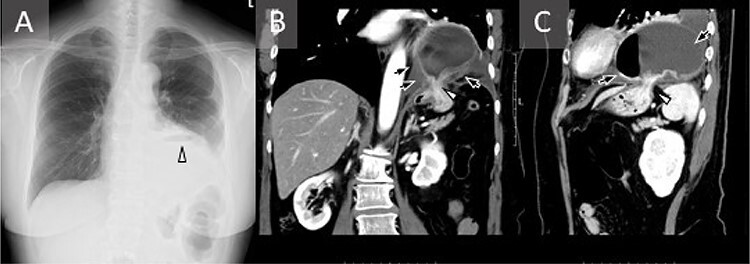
Chest radiography and computed tomography (CT) images obtained 2 days after admission. (A) The gastric bubble is located above the elevated left hemidiaphragm (white arrowhead). (B, C) The contrast-enhanced CT scan shows the hernia (white arrow), with a contrast defect in part of the gastric wall (black arrow).
